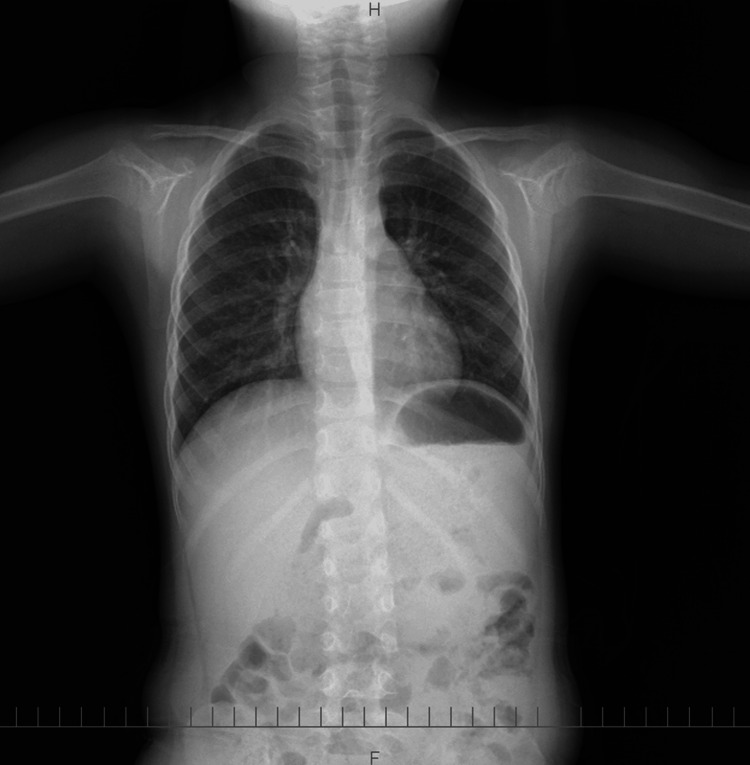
Chest X-ray No obvious foreign body could be identified.
